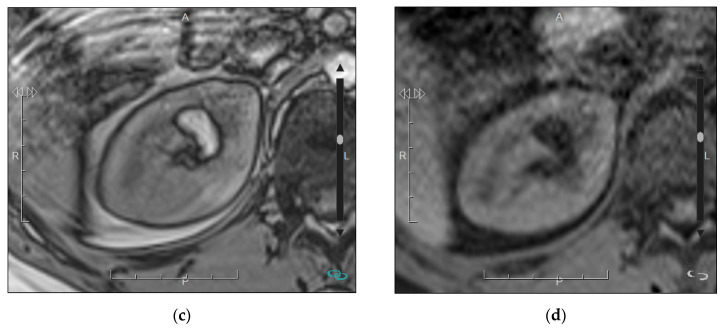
Angiomyolipoma in a 67-year-old woman. (a) Axial T2-weighted image shows a 2.3 cm hyperintense renal mass; (b) axial in-phase (c) and opposed-phase T1-weighted images show India ink artifact on the opposed-phase image; (d) axial fat-suppressed T1-weighted image shows low signal intensity of the mass. Scale size: 5 cm
